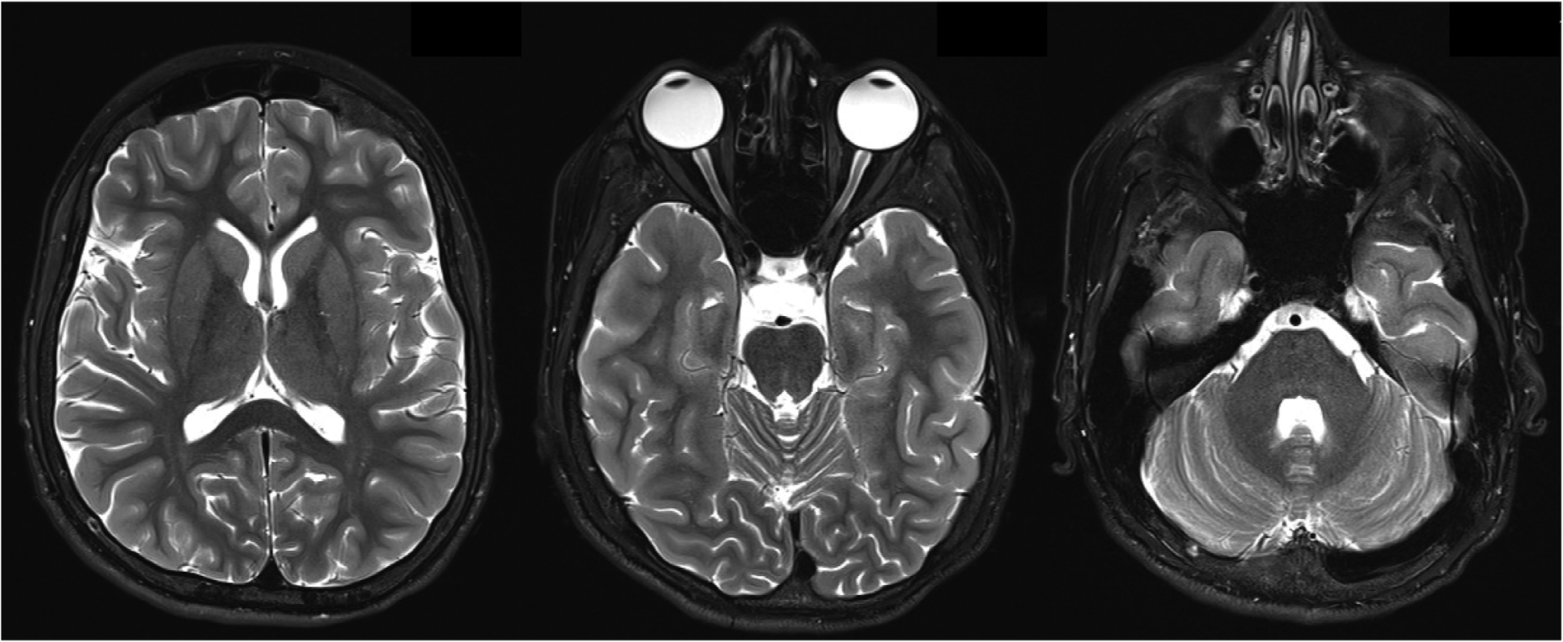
Optic nerve atrophy in patient with biallelic *TSFM* gene mutation. T2 weighted brain MRI images of the patient at the age of 13 years.
